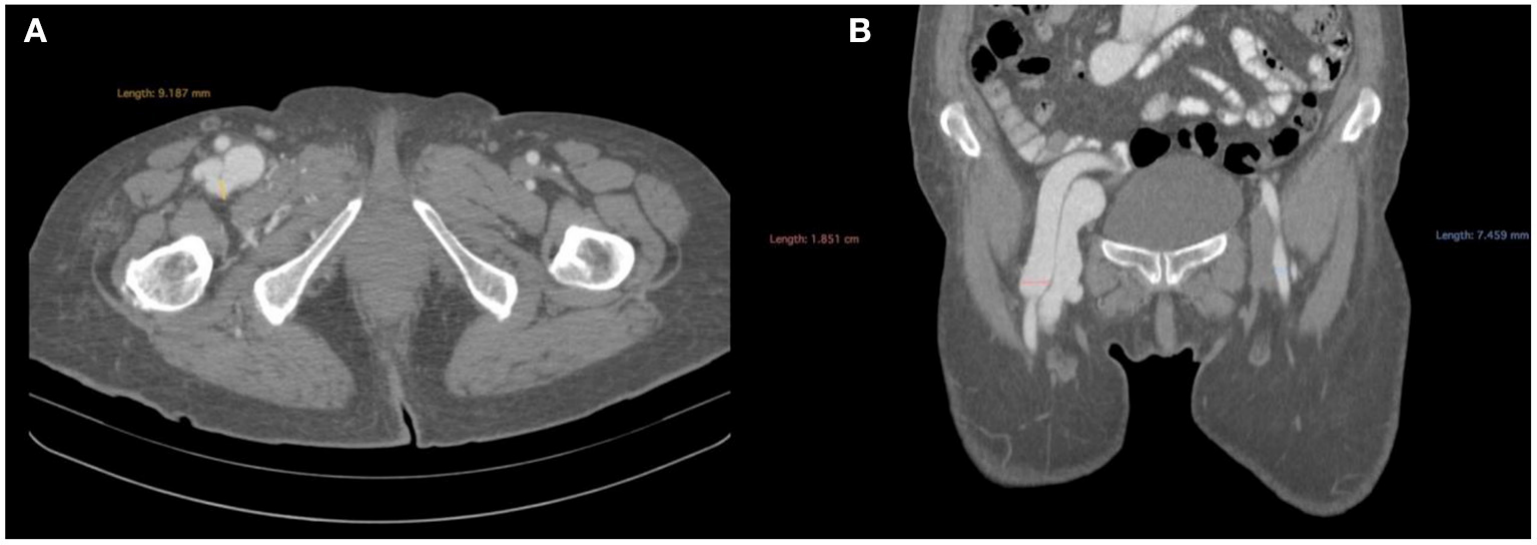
CT angiogprahy before the operation presenting: (A) axial section, femoral artery bifurcation visualization and measurement of the fistula and (B) coronal section, femoral artery bifurcation- visualization of contrast in the femoral vein.
